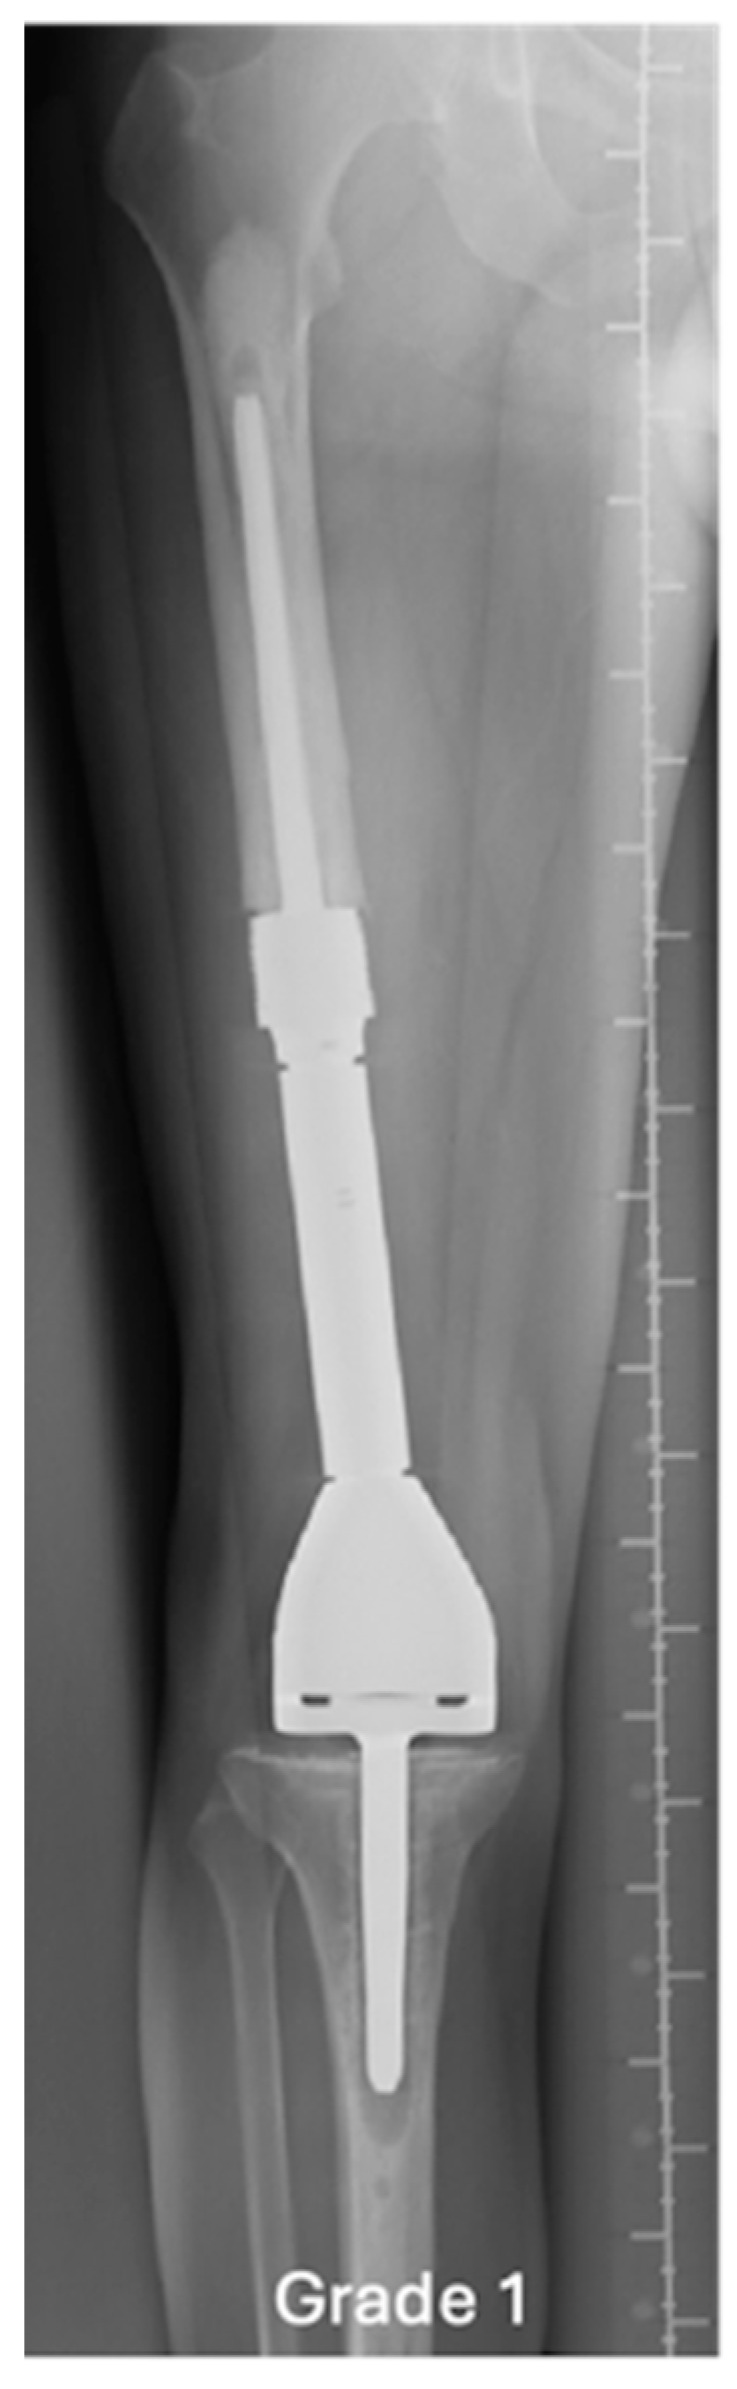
AP radiograph showing normal EPR, grade 1.
